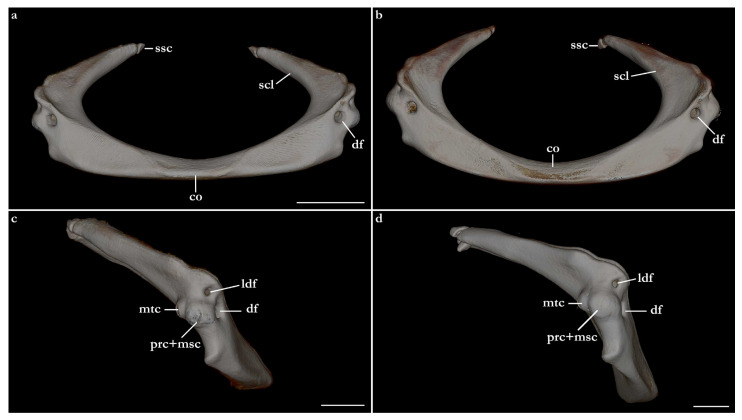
Micro-computed tomography scans of the pectoral girdle of (a,c) *Squatina leae* sp. nov., paratype, ZMH 26098, juvenile male, 249.6 mm TL and (b,d) *S. africana*, ZMH 123064, juvenile female, 309 mm TL in (a,b) frontal and (c,d) lateral views. Abbreviations: co, coracoid bar; df, diazonal foramen; ldf, lateral diazonal foramen; mtc, metacondyle; prc + msc, condyle for propterygium and mesopterygium; scl, scapula; ssc, suprascapula. Scale bars (a): 10 mm, (c,d): 5 mm.
